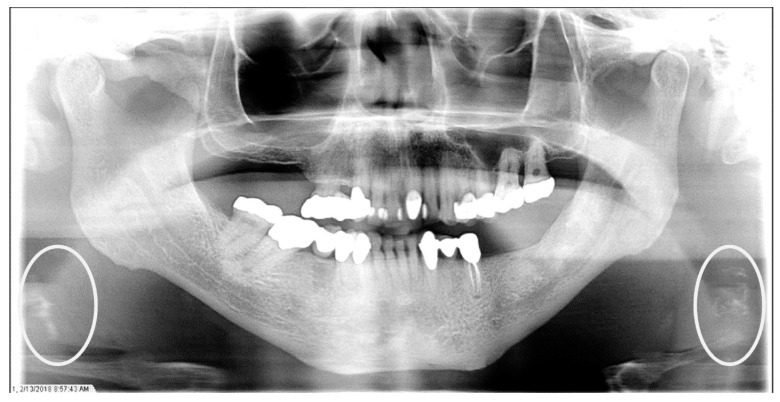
The panoramic radiograph of a 73-year-old male patient with a medical history of hypertension, hyperlipidemia, diabetes mellitus, and cerebrovascular accident showing bilateral carotid artery calcifications (CACs) during a comprehensive dental examination. The CACs are encircled by a white line.
